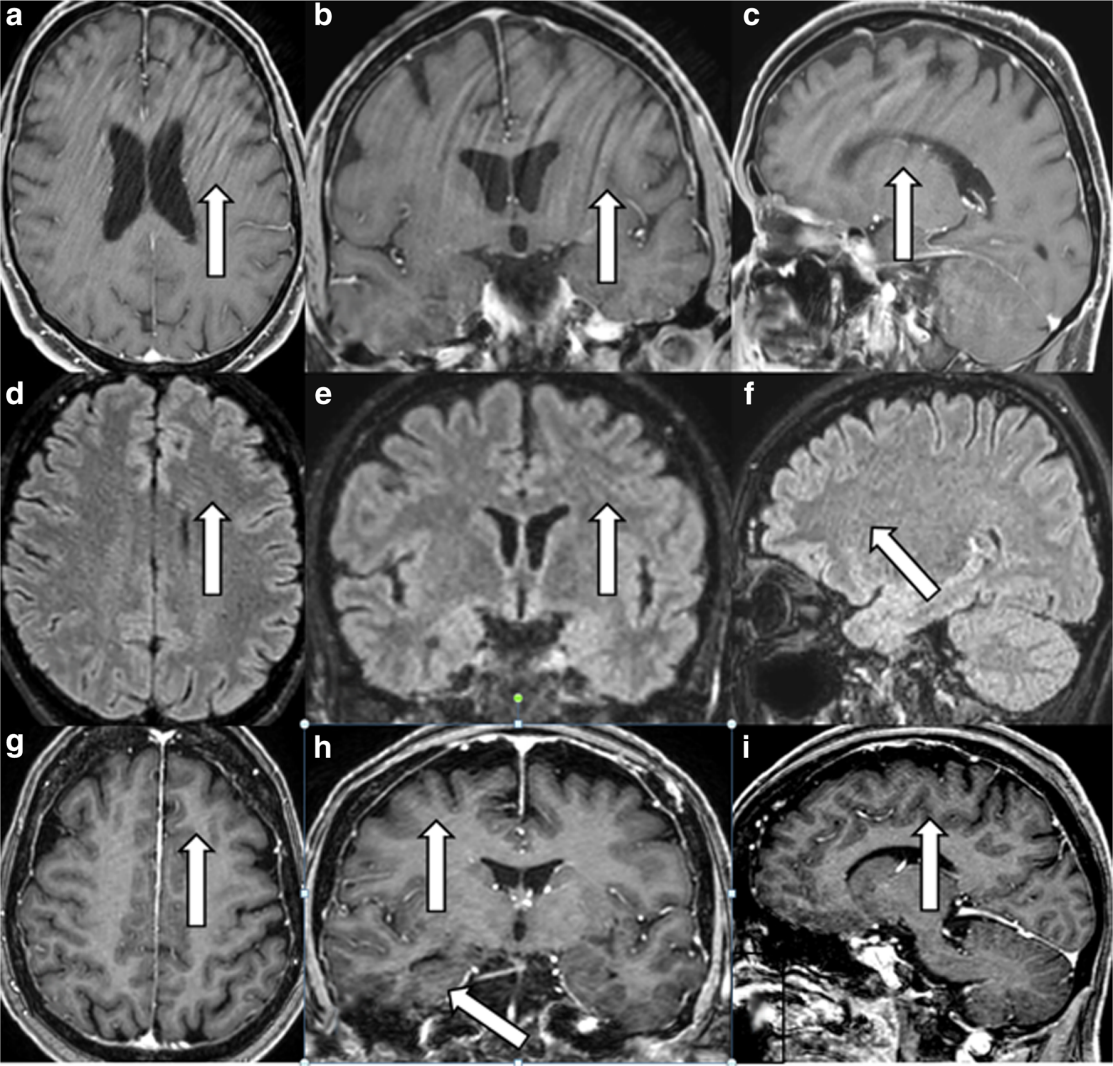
The streaky linear artefact type A. Streaky artefacts type A (*white arrows*) on postcontrast 3D T1 m-Dixon TFE (Fa-c) and on 3D FLAIR (d-f) and on 3D T1 TFE postcontrast sequence (g-i). The streaks are horizontal or oblique, long or short lines, most obvious on reconstructed transverse images (a, d, g) and on reconstructed coronal images (b, e, i). The streaks are less obvious infratentorially or on sagittal source images (c, f, i) where the streaks appear as slightly curved ringing artefacts in various spatial frequencies
